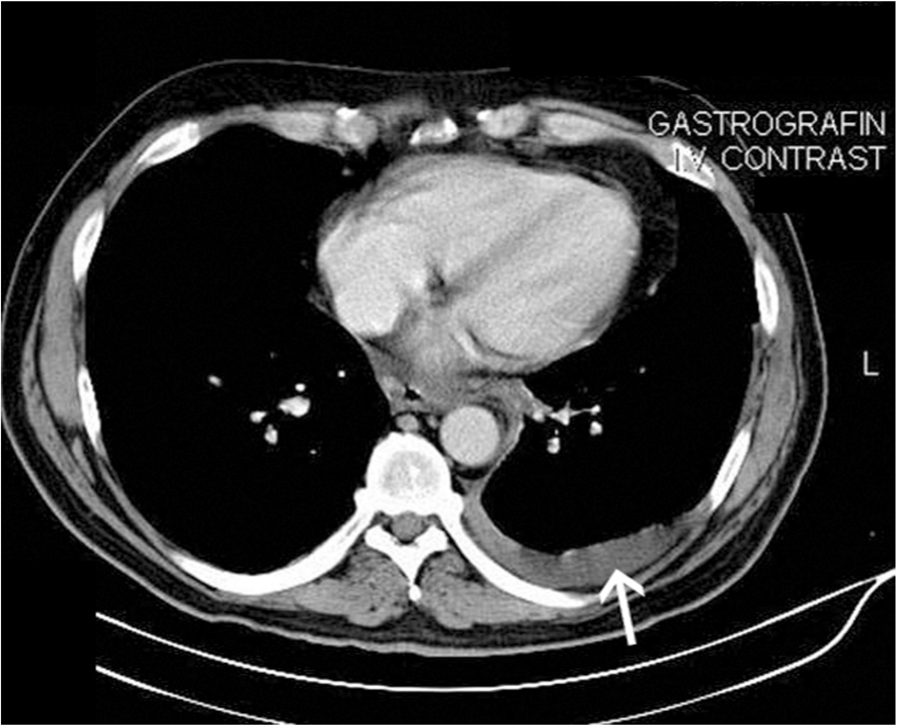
Nine months earlier, an axial post-contrast computed tomography scan reveals no abnormality in the mediastinum. A small pleural effusion is present at the left hemithorax (arrow).
